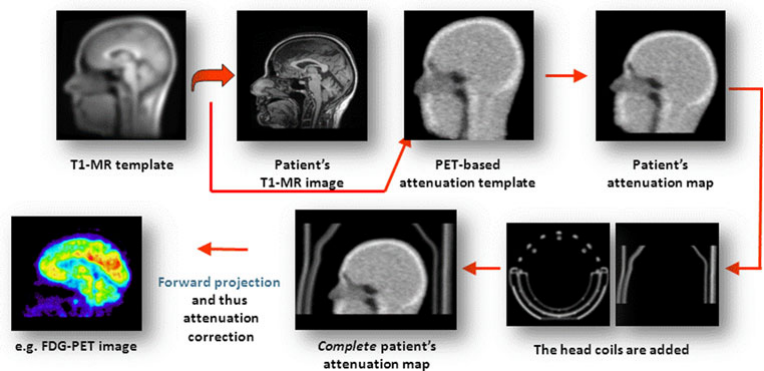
Steps in a template-based attenuation correction approach for brain [14]: The MR template is warped to match the individual MR image using SPM. The obtained transformation matrix is applied to warp the attenuation map template to generate an individualised attenuation map to which the coil attenuation map is added. The attenuation correction factors are obtained by forward projection
